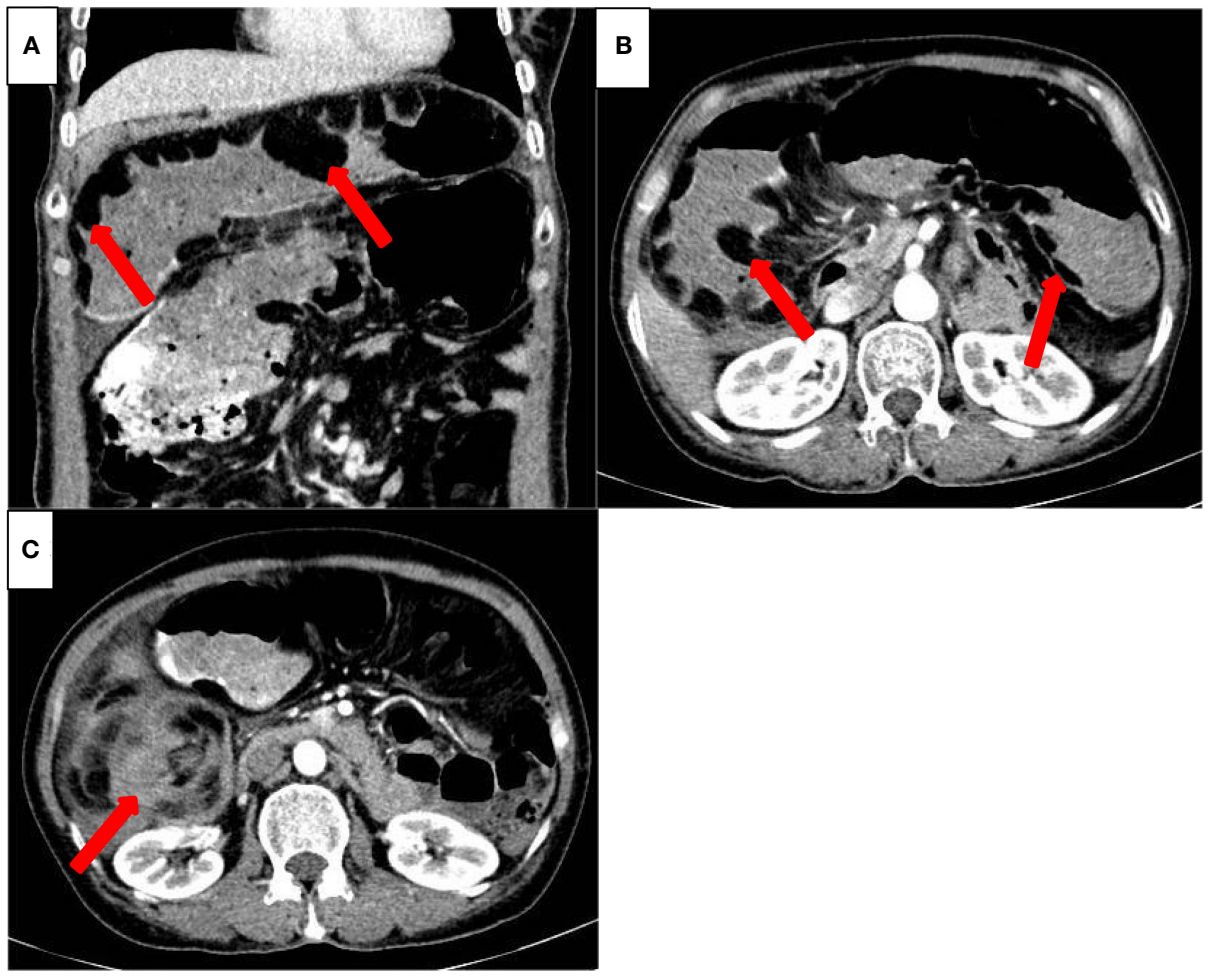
Preoperative three-dimensional enhanced abdominal CT (transverse view (A), coronal view (B) Multiple, irregular lipoid-like density foci (indicated by red arrow) can be seen in the enlarged small intestinal cavity, that showed no enhancement on the enhanced CT scan. (C) The small intestine appears swirling (red arrow).
